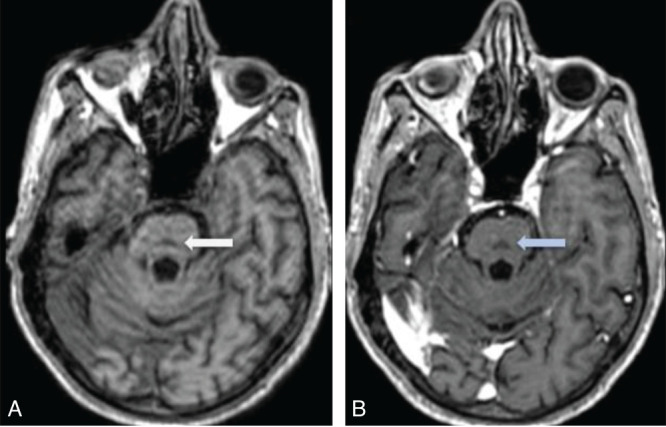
Axial T1 (A) and post-contrast axial T1 (B) images show hypointensity on T1-weighted images, with no contrast enhancement on post-gadolinium images (blue arrow).
